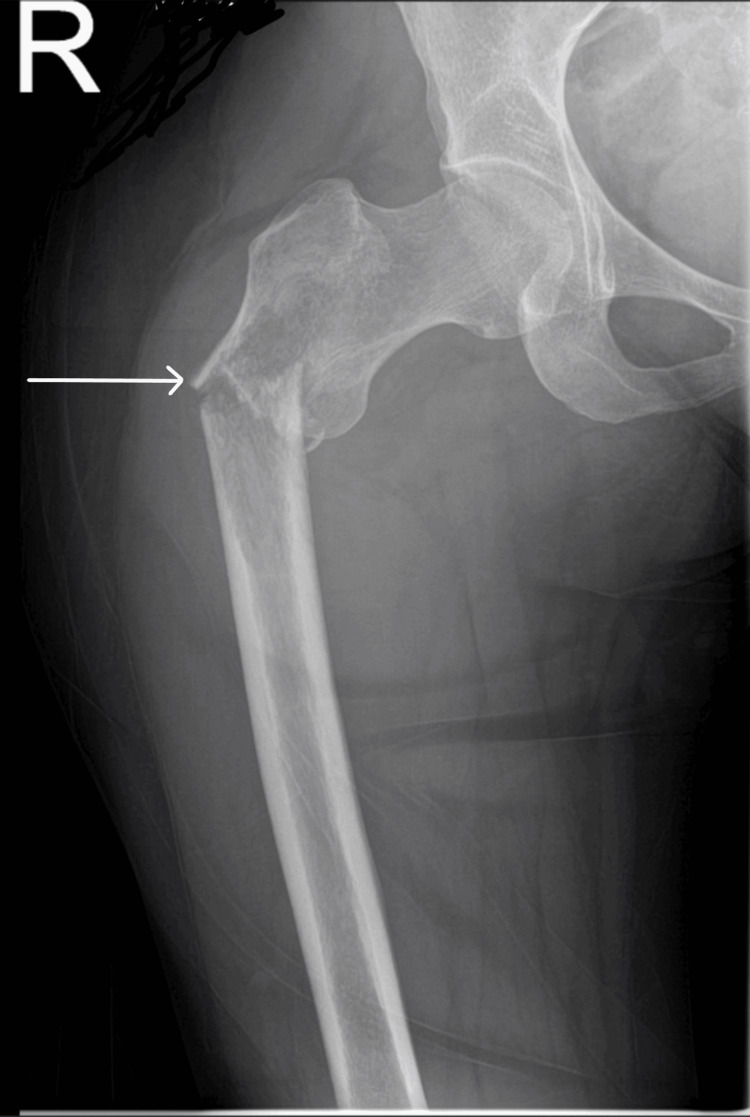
Radiograph of the right hip showing a suspicious lytic lesion
